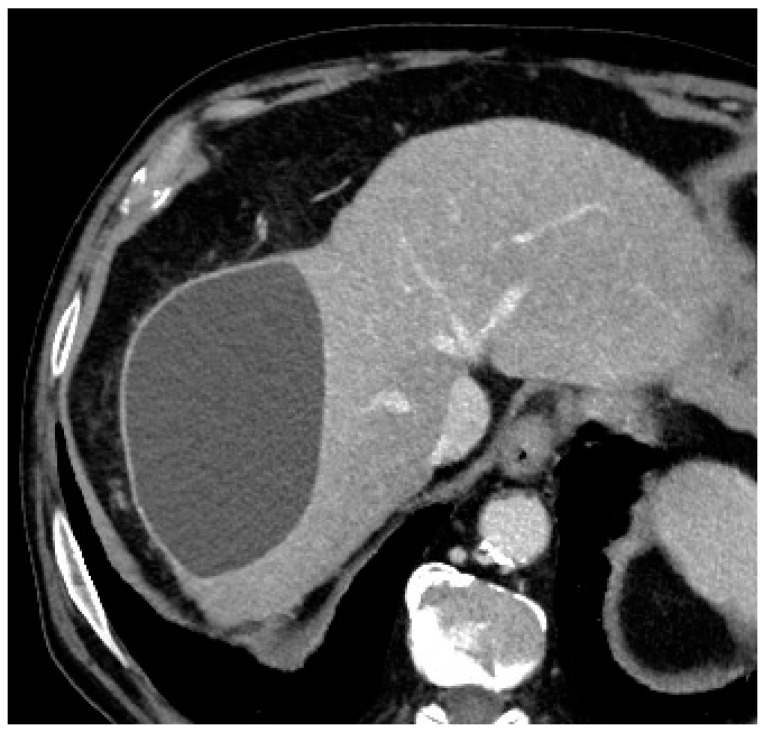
Axial contrast-enhanced CT scan showing an 11 cm × 7 cm × 7 cm biloma in the VII hepatic segment at the 1-month post-procedural follow-up evaluation.
